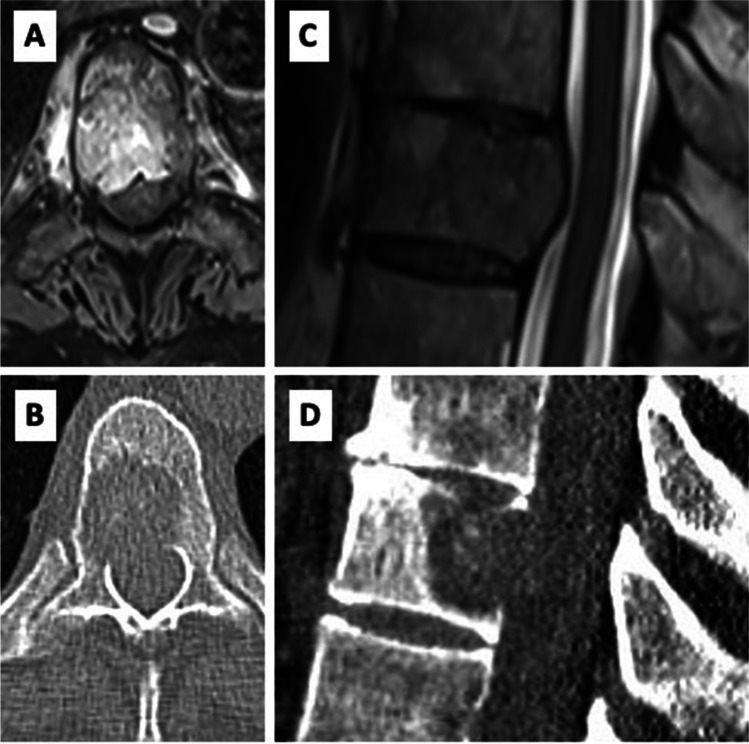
Baseline imaging A) axial MRI T1+Gd B) axial CT C) sagittal MRT T1-Gd D) sagittal CT
